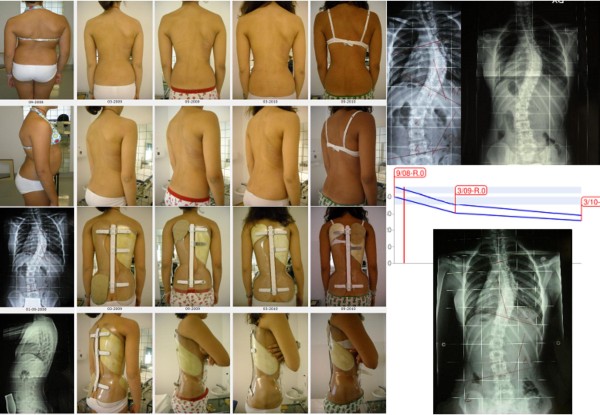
Fourth case report: adolescent double thoracic, lumbar scoliosis patient over 45° still in treatment. On the left: posterior (first line), and sagittal (second line) aesthetics, and the brace in use (posterior - third line; lateral - fourth line) of all evaluations (apart the first visit, where aesthetics and first x-rays are shown) are reported from left (oldest) to right (last one). On the right, top-down, left-right direction: all x-rays of this case report of a patient still in treatment, and the graph with obtained results in the two curves: upper line, thoracic curve, lower one lumbar curve. C.F. has been evaluated the first time in September 2008, presenting with the first x-ray showing a thoracic right lumbar left scoliosis of 46°-39° (Risser 0). She started treatment with the Sforzesco brace 23 hours per day and SEAS exercises 2-3 times a week (45' per session): after 6 months, while growing 5.5 cm. (from 158.5 to 164), she was 36°-31° (Risser 0). Treatment continued 6 more months 23 hours per day, then reduced to 22: in this year, while growing 4 cm. (to 168), she reduced her scoliosis to 29°-27°. After 6 months at 20 hours, now she is wearing the brace 18 hours per day.
